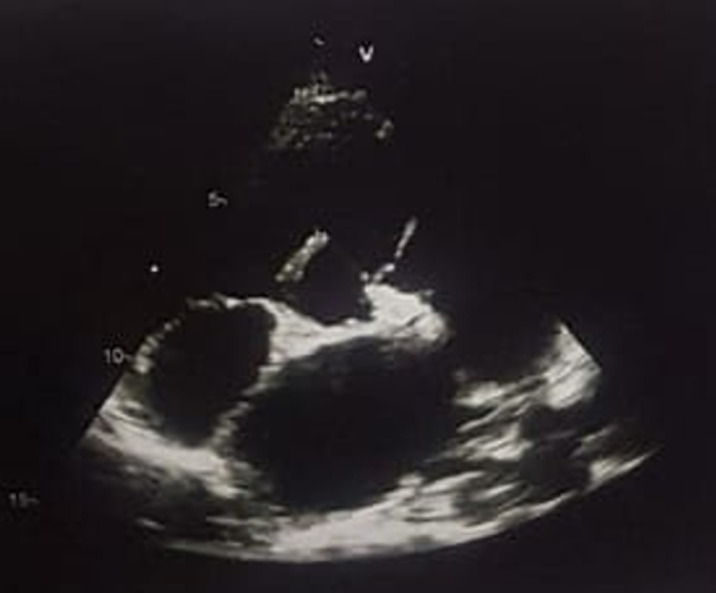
transthoracic echocardiography showing ventricular wall defect with dilatation of the four cardiac cavities
